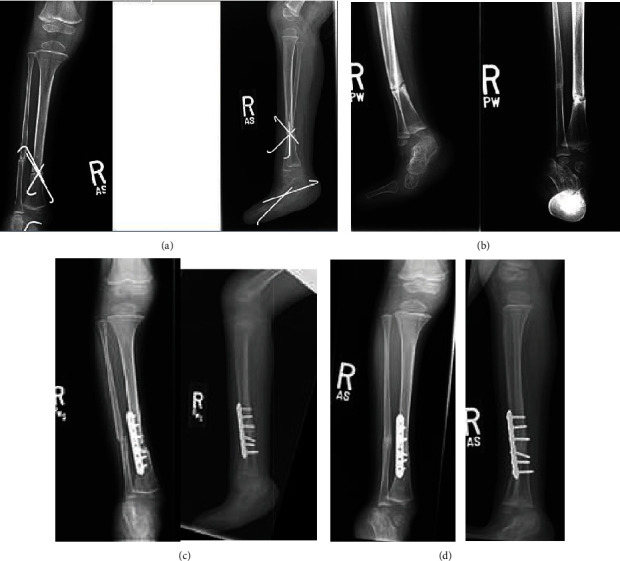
Supramalleolar derotational osteotomy in a 3-year-old male fixed with percutaneous 0.062-inch Kirschner wires. (a) Anteroposterior and lateral radiographs at 9-week postoperative time point demonstrating delayed healing of the tibial osteotomy site. (b) Six-month postoperative radiographs demonstrating no interval evidence healing at the tibial osteotomy site. (c) Immediate postoperative radiographs following revision open reduction with internal fixation of tibial nonunion. (d) Three-month postoperative radiographs showing union of the tibial osteotomy site.
